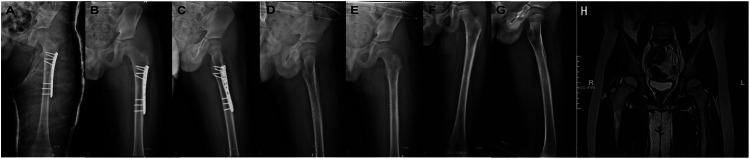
Postoperative radiographs and magnetic resonance imaging of the left hip and thigh. (A) Radiograph on postoperative day 2 showed an adequate reduction of the hip joint and subtrochanteric fracture with an excellent implant placement. (B–E) Fracture healing was observed before and after the removal of internal fixation (16 months after the primary surgery). (F,G) No signs of osteonecrosis, early closure of the epiphyseal plate, heterotrophic ossification, and osteoarthritis were observed on the anteroposterior and lateral radiographs at 3 years of follow-up after injury. (H) MRI of the hip at 3-year follow-up after injury showed no signs of osteonecrosis.
